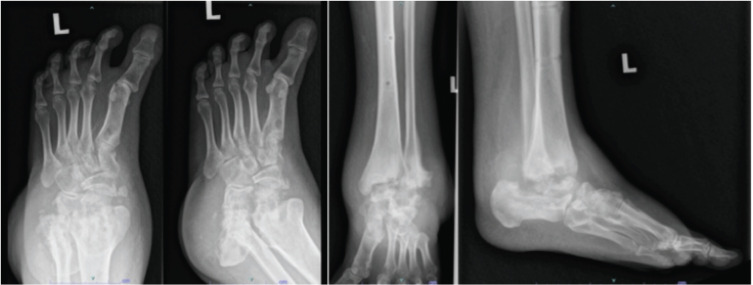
Radiographs image before starting pamidronate infusion treatment.
